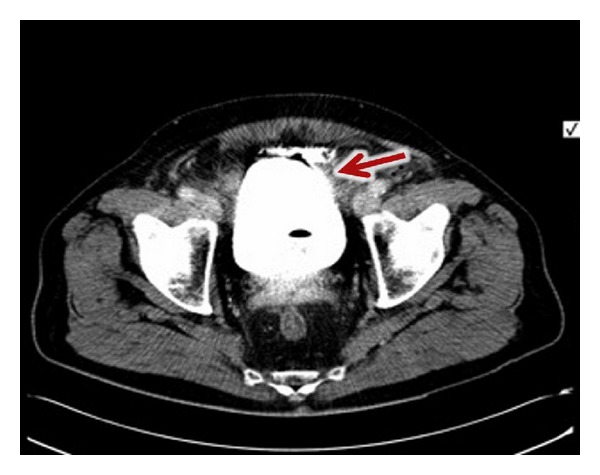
Computed tomography with retrograde cystography (axial view) showing extraperitoneal bladder perforation along anterior wall (arrow points to extravasated contrast).
